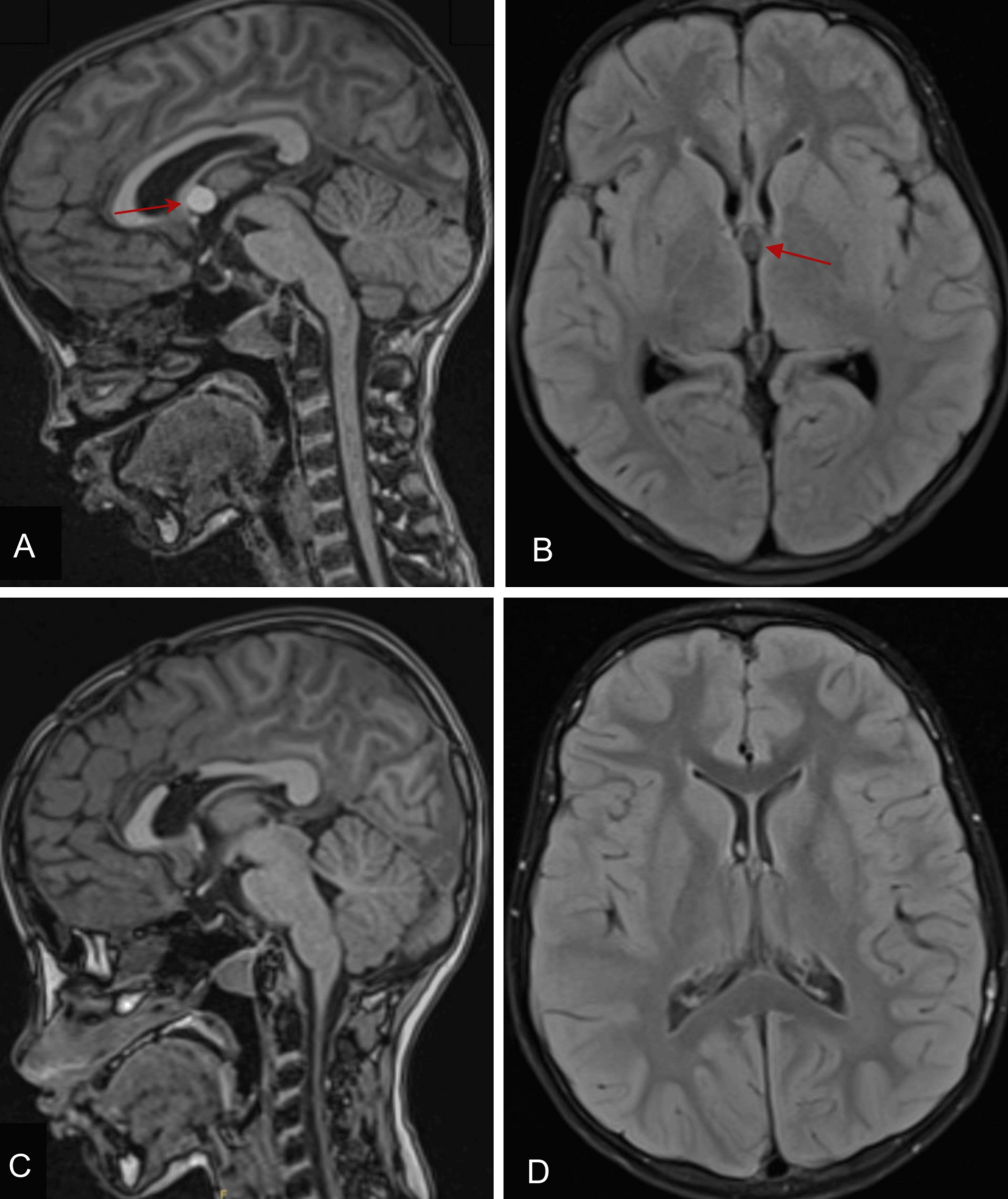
Preoperative MRI demonstrating a third ventricular colloid cyst, and postoperative MRI confirming complete resection. (A) Preoperative sagittal T1-weighted MRI showing a cyst that is hyperintense to the CSF within the third ventricle (red arrow). (B) Axial preoperative view. (C) Postoperative sagittal T1-weighted MRI showing the successful resection of the colloid cyst and restoration of the CSF flow. (D) Axial postoperative view. CSF, cerebrospinal fluid; MRI, magnetic resonance imaging.
